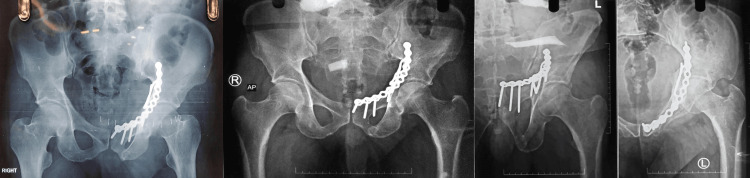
Postoperative radiographs A) Postoperative pelvis anterior-posterior (AP) radiograph; B) Follow-up pelvis anterior-posterior radiograph at six months C) Follow-up pelvis outlet view radiograph at six months D) Follow-up pelvis inlet view radiograph at six months.
